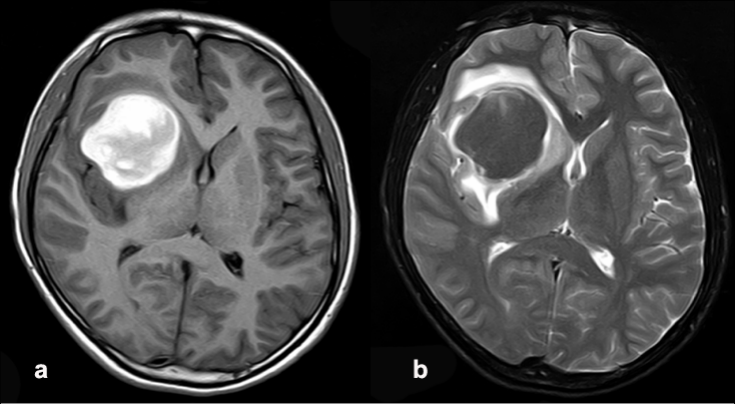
Figure 1:(a) Post-contrast T1-weighted image demonstrating heterogeneous enhancement of the lesion. (b) T2-weighted image demonstrating hypointense lesion in the right insular lobe with surrounding edema and hematoma.
